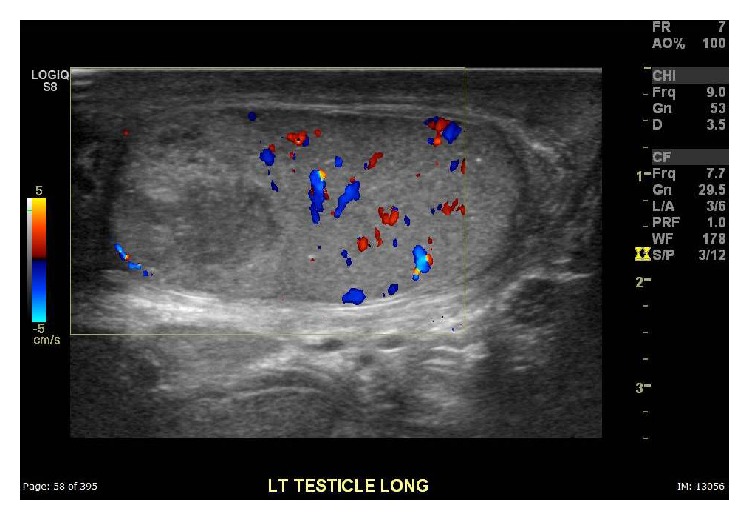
Follow-up color-Doppler ultrasound image shows interval enlargement of the testicular lesion.
